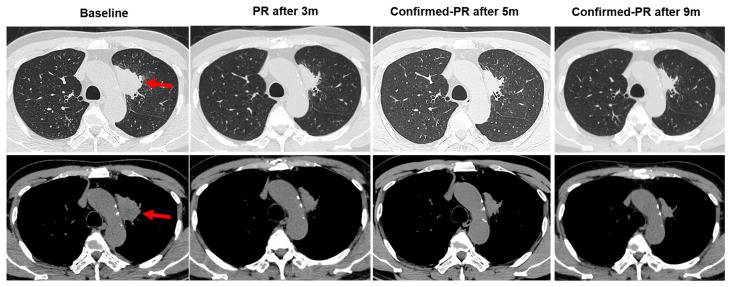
Changes in primary lesions on chest CT images pre- and post-entrectinib therapy. The chest computed tomography (CT) scan images showing the location of tumor lesions found in the left upper lung (red arrows). After taking entrectinib, 600 mg/day, orally for 3 months, the tumor showed a decrease in size; oral administration of entrectinib for 5 months indicated the tumor continued to shrink in size; oral administration of entrectinib for 9 months showed that the tumor was continuously reduced in size. PR: partial response.
